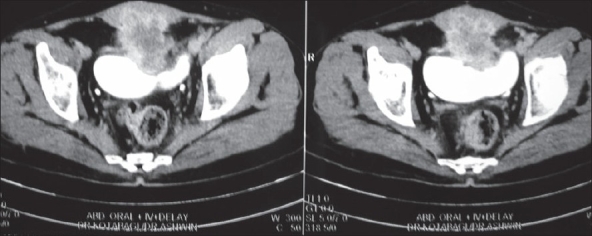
CT scan demonstrating anterior bladder wall growth infi ltrating recti muscles
